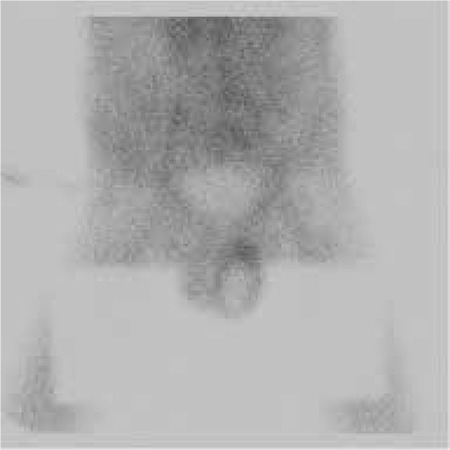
The anterior static view of testis scintigraphy showing hypoactive left testicular area surrounded by an hyperactive halo.
